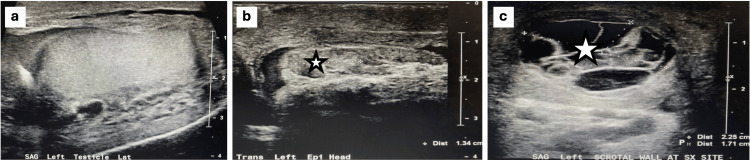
Scrotal ultrasound images upon presentation (a) Lateral left testicle. (b) Trans view of left epididymis head. (c) Left scrotal wall view at surgery site. The star represents the surgical site. All scale bars represent centimeters.
